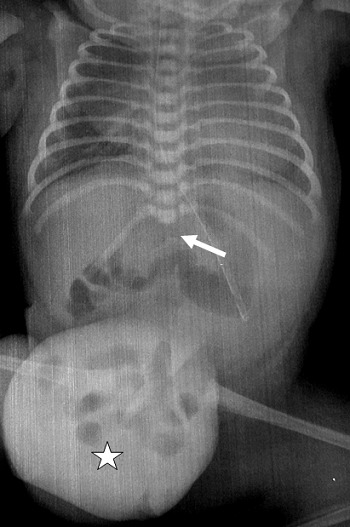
Postnatal thoracoabdominal X-ray: there are 12 dorsal vertebrae of normal morphology, with total agenesis of the lumbosacral spine (arrow); the omphalic sac containing digestive clarity
